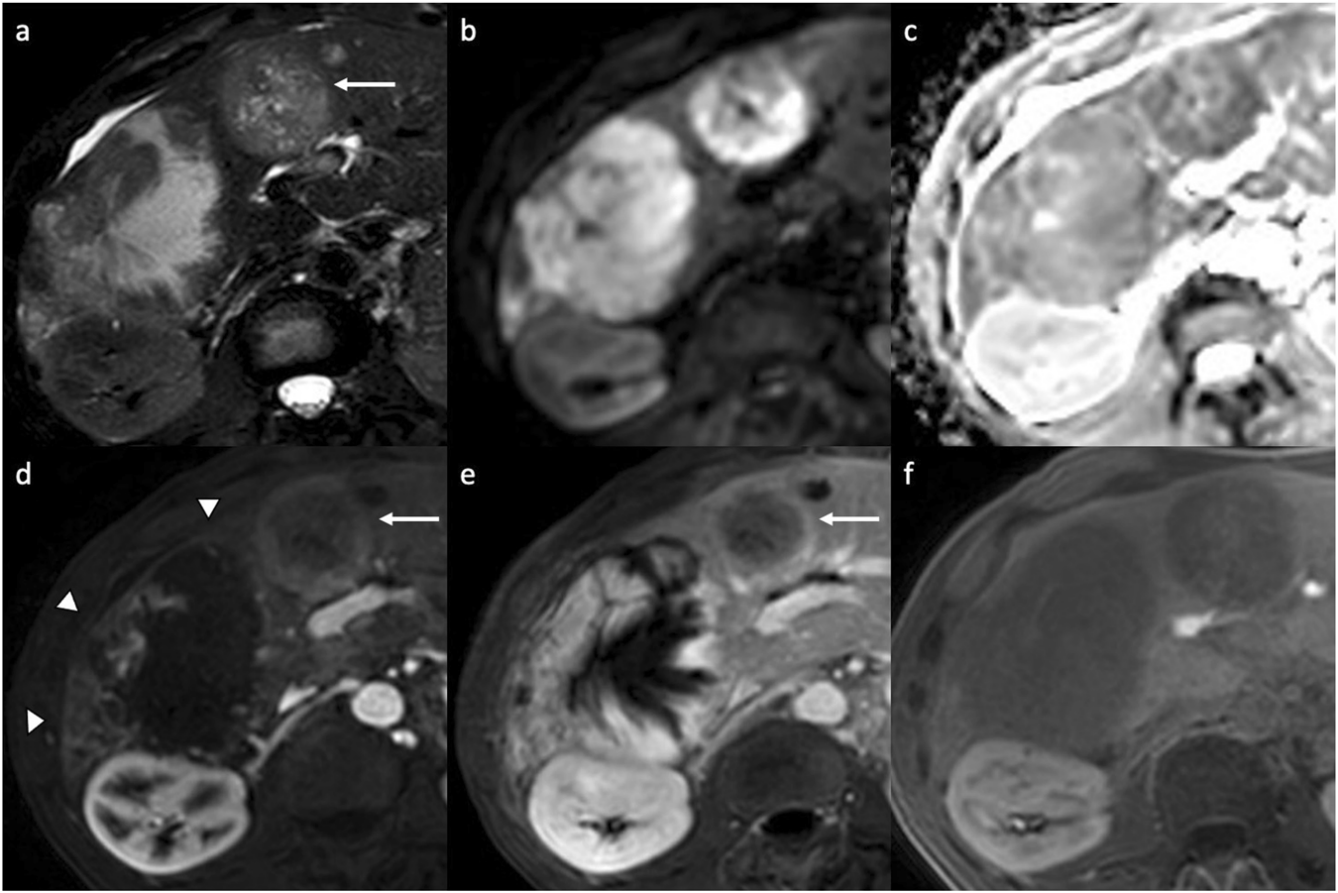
HCC and cavernous hemangioma in a 45-year-old man. a Axial T2-weighted MRI shows a subcapsular lesion with slightly high SI (arrow), with high SI on (b) DWI and low values on (c) the ADC map. d On gadobenate dimeglumine-enhanced MR sequences, the lesion shows a rim arterial phase enhancement (arrow), (e) capsule appearance in the delayed phase, and (f) low SI in the hepatobiliary phase, suggestive of HCC. Contrast-enhanced MRI also shows a large lobulated mass located in the right hepatic lobe, with high SI on (a) T2-weighted images, (b, c) without diffusion restriction, and with peripheral discontinuous nodular enhancement on (d) AP (arrowheads), followed by progressive centripetal enhancement on (e) DP (cavernous hemangioma)
